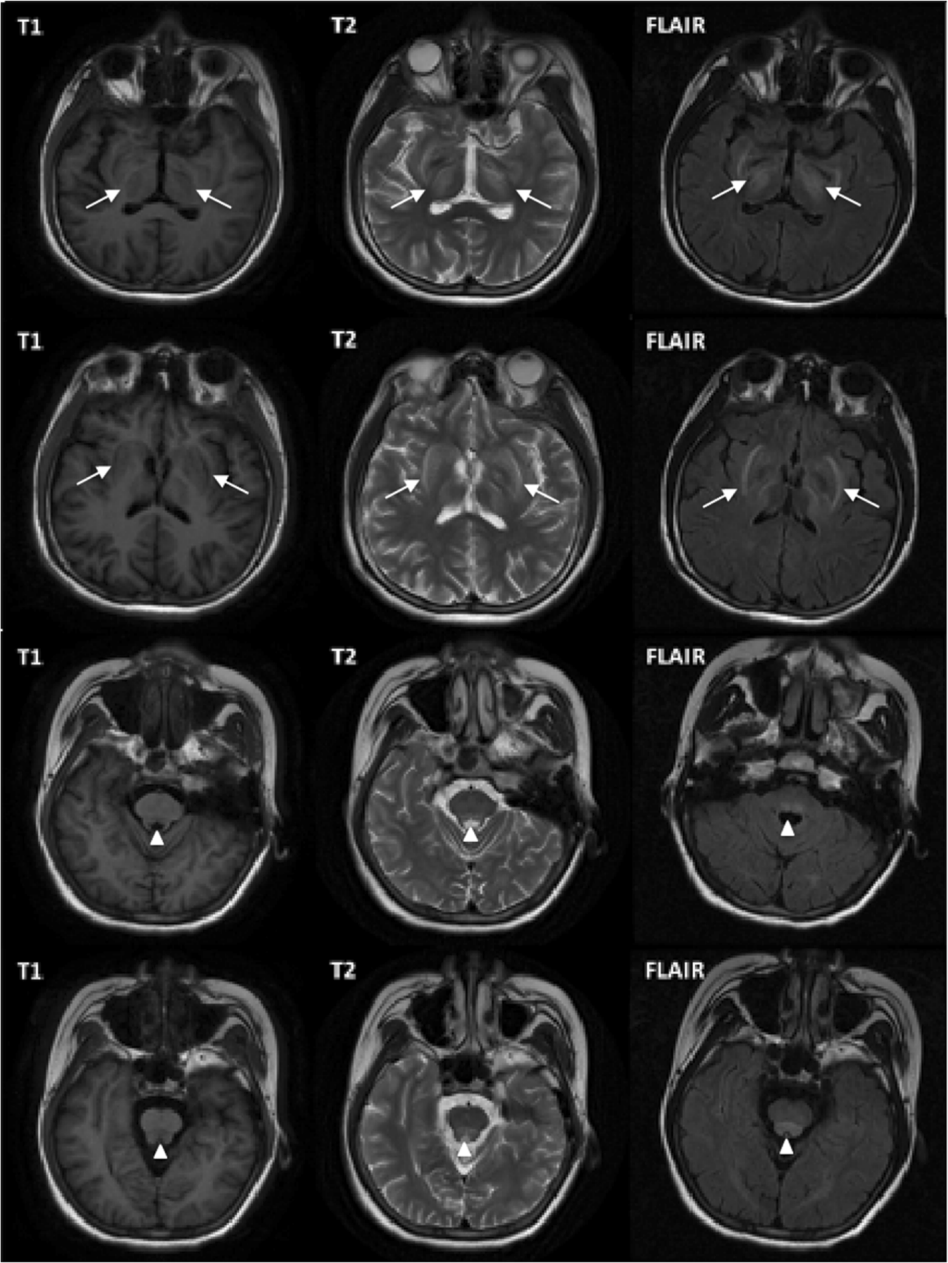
MRI of the patient showed symmetric abnormal signals with low signal in T1-weighted image, and high signals in T2-weighted and FLAIR images of bilateral basal ganglia, thalamus, midbrain, and pons
